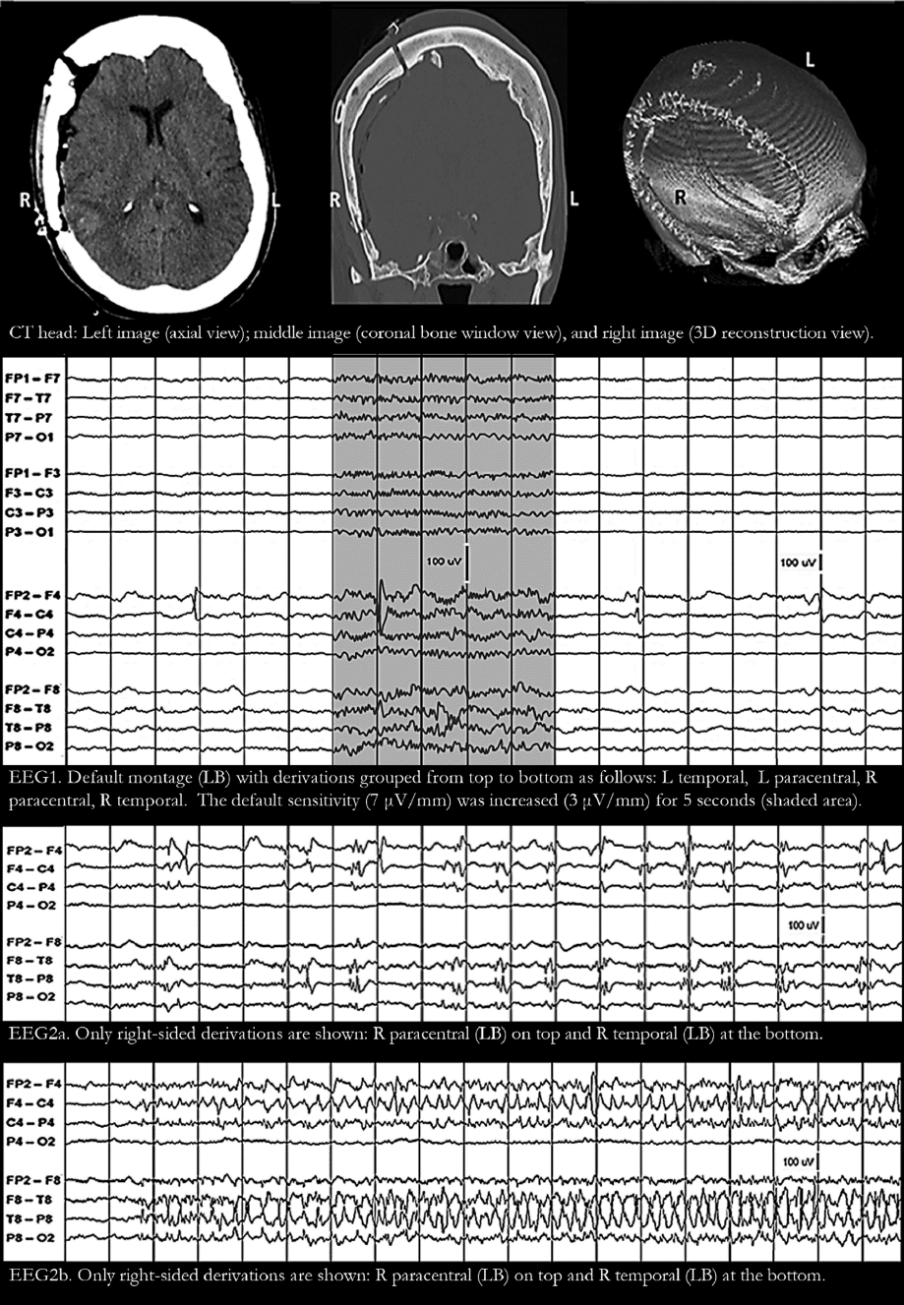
Patient 3 has a large right frontotemporal craniectomy with bone flap in place (CT [computed tomography] head). Electroencephalogram (EEG) showed intermittent F4 potentials with epileptiform morphology but no physiological field suggesting F4 electrode artifacts (EEG1). Increasing display sensitivity showed a hint of a physiological field but this was not enough to rule out electrode artifact (EEG1 shaded area). Adding 10-10 electrodes was not necessary since focal periodic epileptiform discharges (EEG2a) and focal seizures (EEG2b) were subsequently recorded in F4 and T4. For all EEG tracings above: filters at 1-Hz high-pass, 70-Hz low-pass, and 60-Hz notch; 1 second/division; and 100-µV voltage scale shown in tracing. L, left; R, right; Ref, reference; LB, longitudinal bipolar; TB, transverse bipolar; LR, longitudinal referential; TR, transverse referential.
